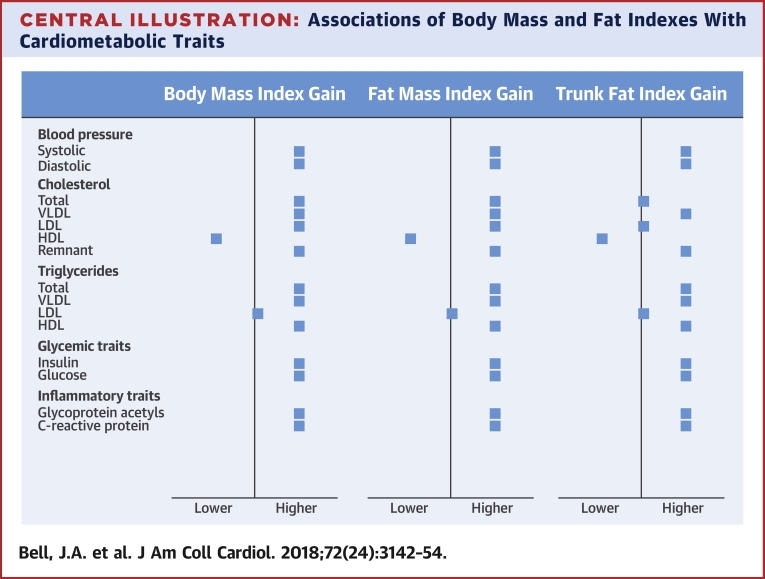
Associations of Body Mass and Fat Indexes With Cardiometabolic Traits Gains in body mass index and dual-energy x-ray absorptiometry–derived total and regional (trunk, arm, leg) fat indexes from 10 to 18 years of age were examined in relation to cardiometabolic traits from clinical assessments and targeted metabolomics at age 18 years among 2,840 offspring from the ALSPAC (Avon Longitudinal Study of Parents and Children) cohort. Models were adjusted for age, sex, ethnicity, maternal education, and index level at age 10 years. Trunk fat index gain was additionally adjusted for change in arm and leg fat indexes. Associations were similar in direction and magnitude for the 3 indexes shown and were weaker for gains in arm and leg fat indexes. HDL = high-density lipoprotein; LDL = low-density lipoprotein; VLDL = very-low-density lipoprotein.
